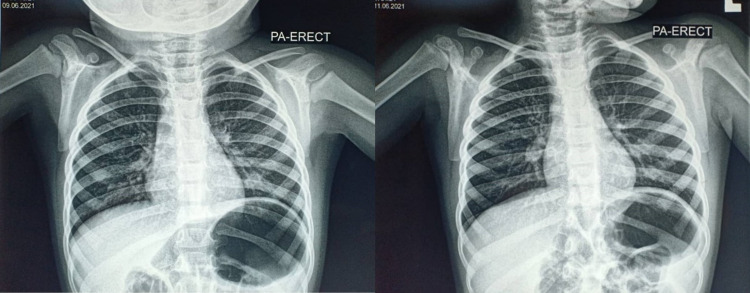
Chest X-ray (PA erect view) taken: clear lung fields and no foreign body seen PA: posterior-anterior
